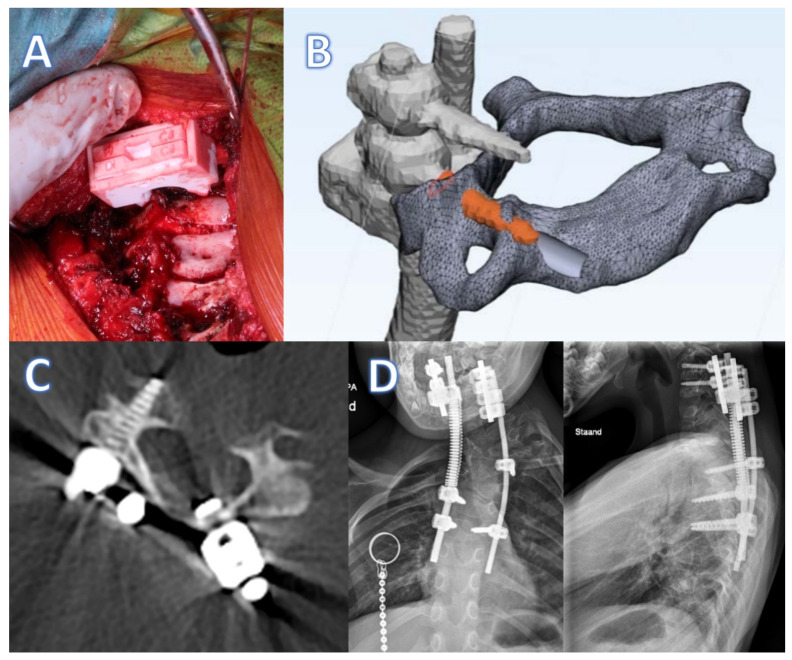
Click-on guide case 1. (A) Intraoperative use of the click-on guide, exposure of the operative site limited due to interference with the posterior skull. (B) Slight upward deviation of the C6 screw (orange) in comparison to the planned trajectory (gray). (C) Post-operative axial CT reconstruction with the screw precisely through the pedicle. (D) Post-operative radiographs of the instrumented spine.
